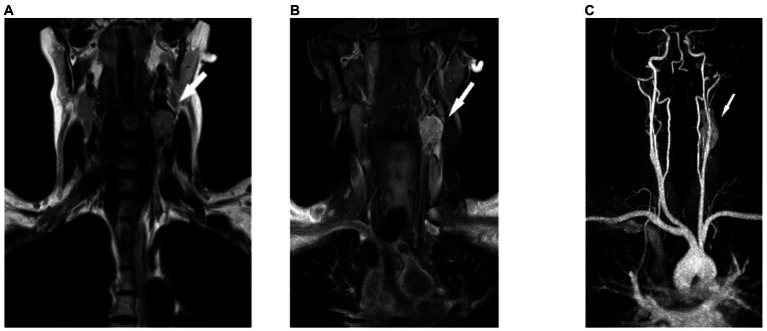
Carotid body tumor on the left side. (A) MR T2-weighted coronal image. (B) MR T1-weighted coronal image with fat suppression after contrast medium administration. (C) Contrast-enhanced MR angiography (CE-MRA), and maximum intensity projection (MIP) reconstruction in the coronal plane.
